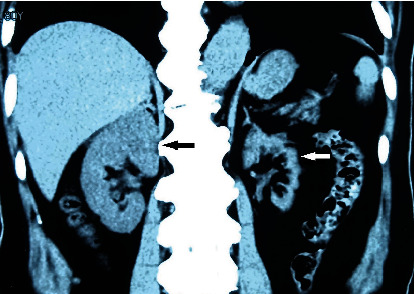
CT image in 2015 shows left renal atrophy, renal cortex thinning (white arrow), and right kidney compensatory hypertrophy (black arrow).
